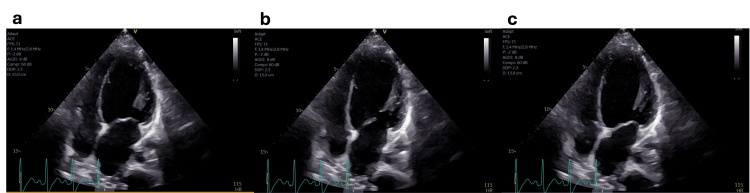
Echocardiography (ECHO). ECHO showed characteristic transient ballooning of the left ventricle, including the apex, with an ejection fraction of 20% and diffuse hypokinesis with sparing of a hyperdynamic apex. (a) Diastole before atrial kick. (b) Diastole after atrial kick. (c) Systole.
